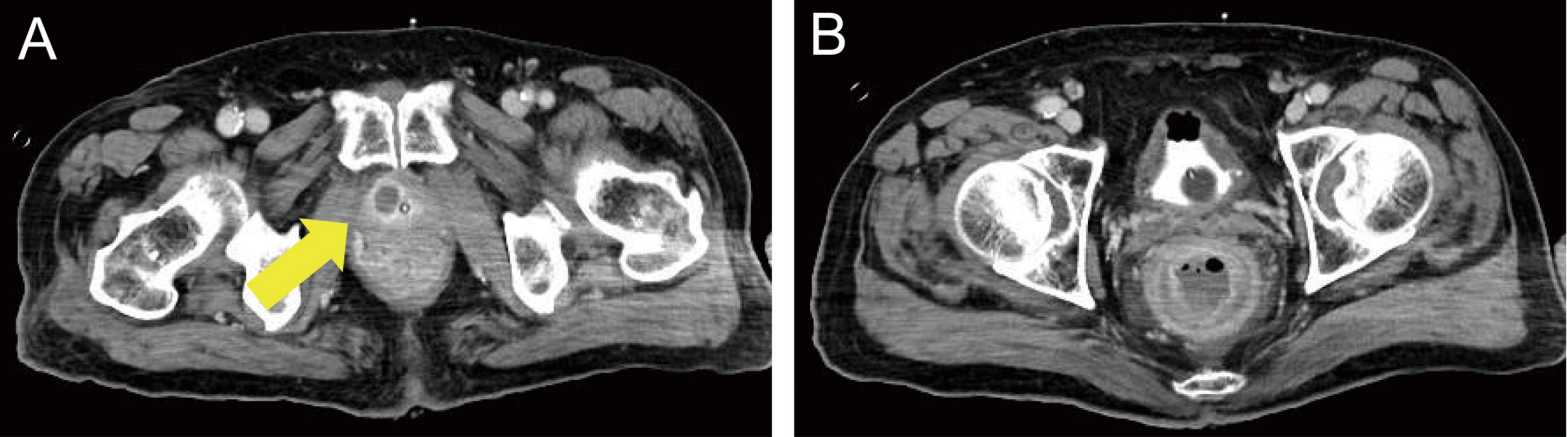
Contrast-enhanced CT performed on day 2 showing (A) a low-density area with a contrast effect seen surrounding the 15 × 12 mm region (arrow) and (B) the fecal mass expelled after which edema and a visible contrast effect were noted in the rectal wall CT: computed tomography
